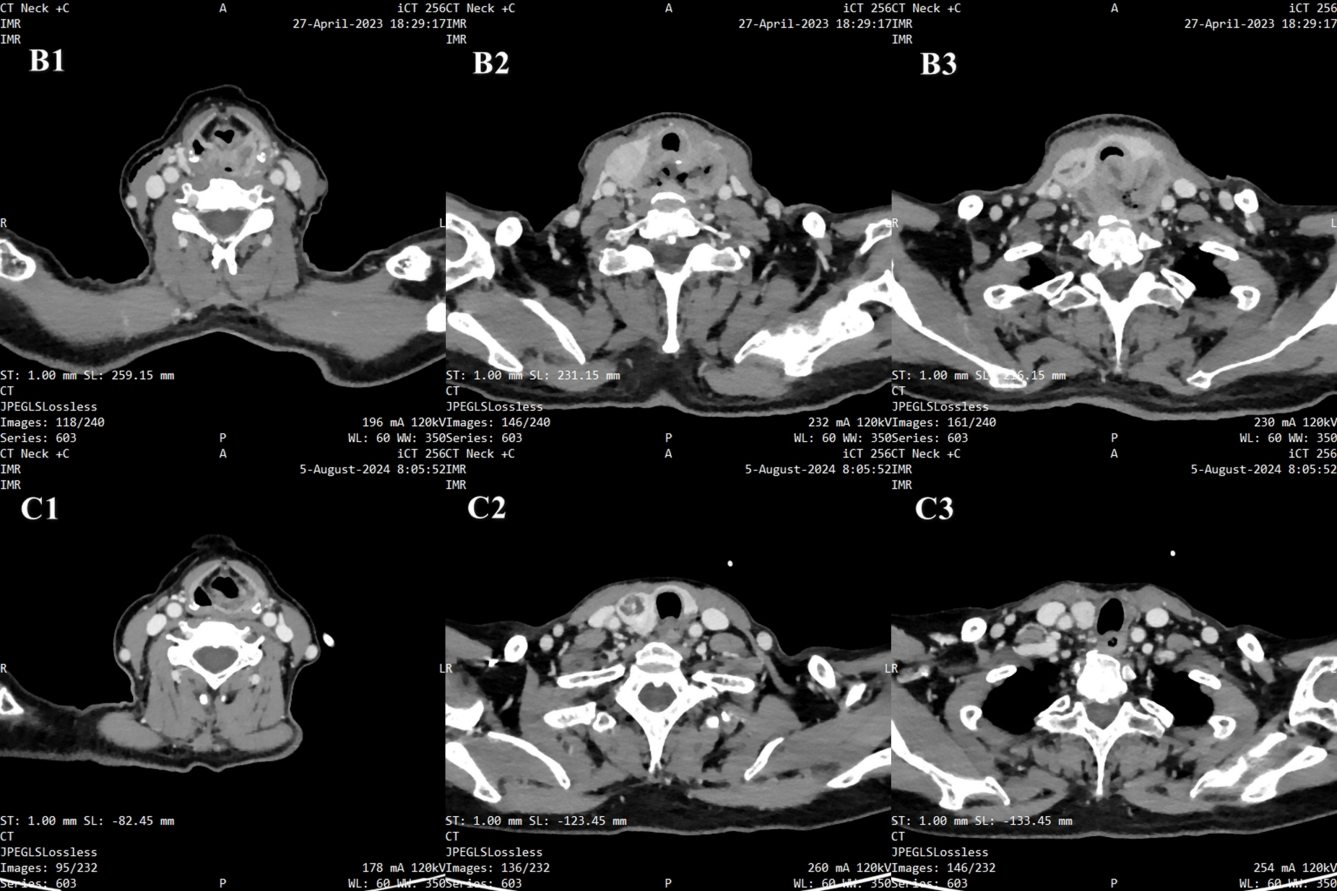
Radiographic images. B1-B3. Pretreatment: A large mass in the hypopharynx and cervical esophagus, with the larynx, trachea, thyroid, and prevertebral fascia involved. C1-C3. Posttreatment: The number of tumors in the hypopharynx and cervical esophagus significantly decreased.
